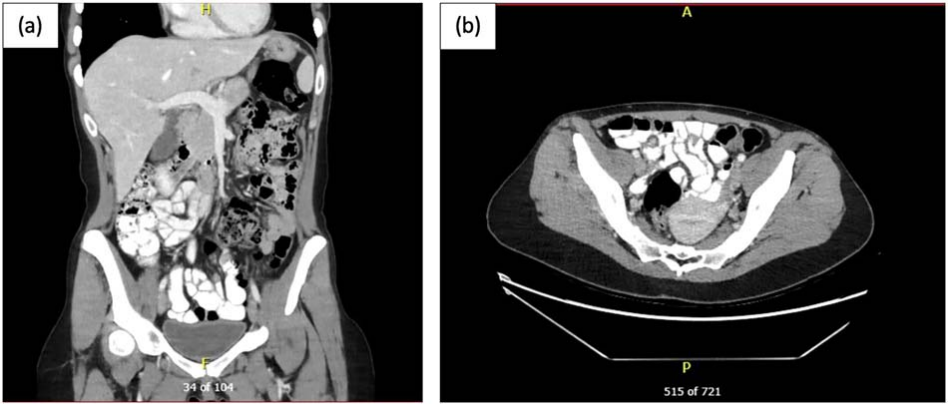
CT AP post oral and intravenous contrast; (a) coronal view and (b) axial view showing multiple mildly dilated small bowel loops, suspicious for developing SBO, with transition point in the right lower pelvis, where there is possible focal mural thickening, and no perforation; the appendix appeared normal.
